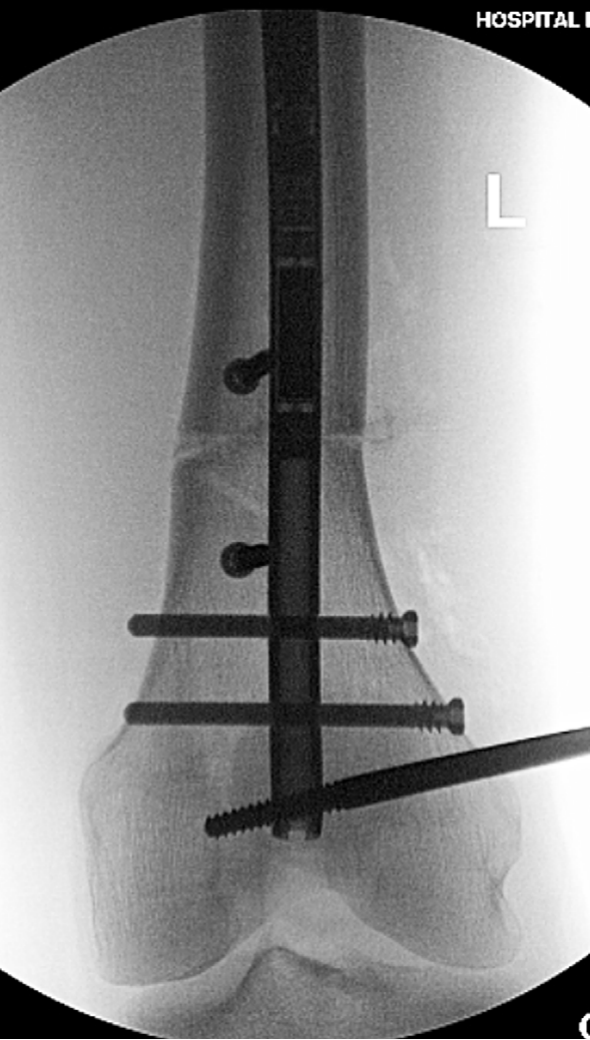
The AP fluoroscopy shot shows the distal femur after successful distal interlocking with the varus deformity corrected. The peri-osteotomy blocking screws are positioned to prevent varus deviation during lengthening. The external half pin is also seen in the field.Follow-up: Lengthening begins after a latency period and proceeds at approximately 1 mm per day. The patient is followed at regular intervals with radiographs checking for regenerate quality and joint contractures (Figures 7a and 7b).Figure 7. F7:
